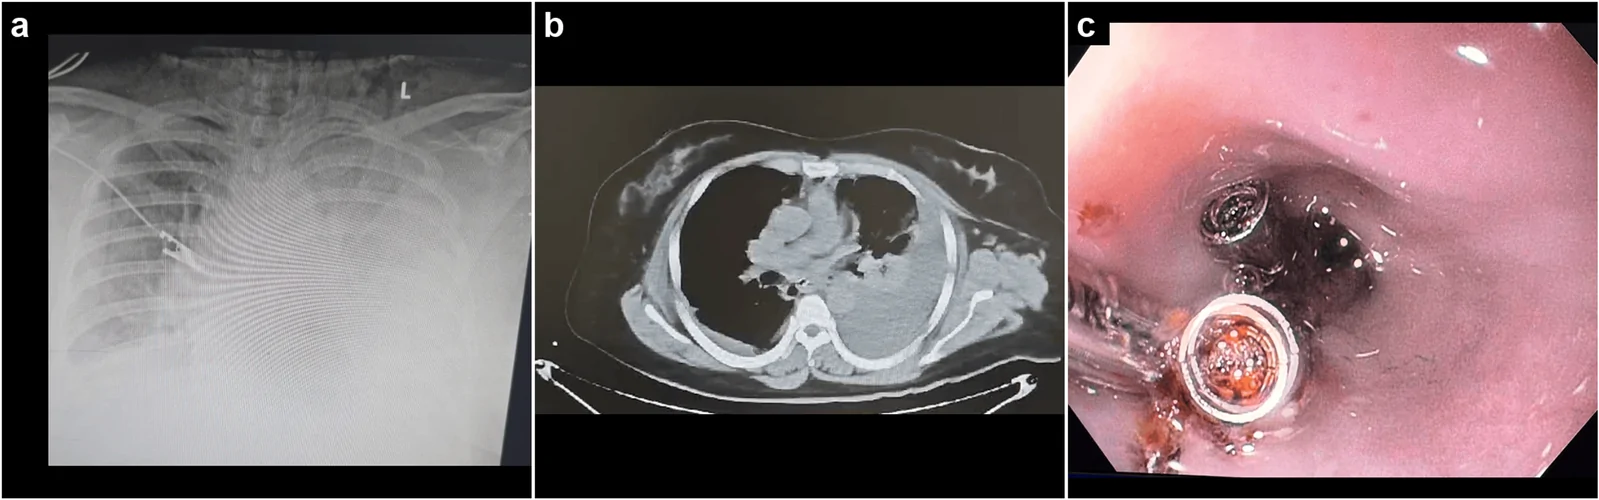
Case 2 Images (a) Chest radiograph showing a massive left pleural effusion. (b) Axial computed tomography showing pneumomediastinum and left pleural effusion. (c) Endoscopic view of the distal-oesophageal perforation with an applied clip.
